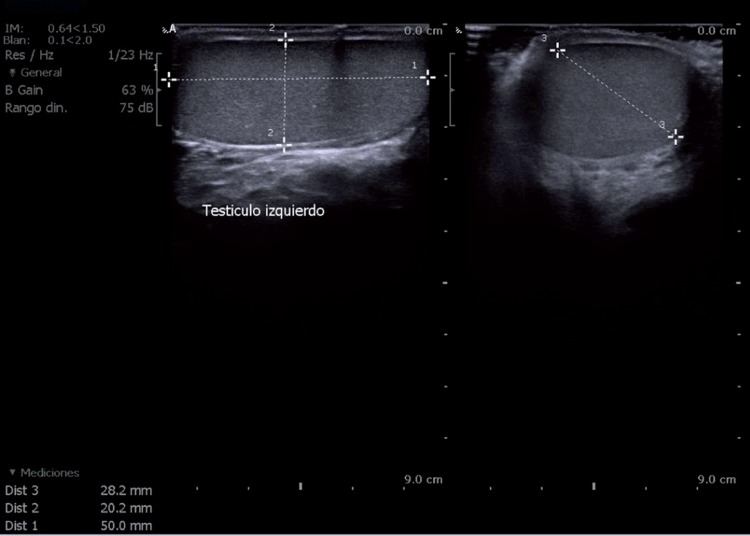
Ultrasound image one year after surgery. Right testis preserves a normal volume (14.8cc) and echogenicity.
